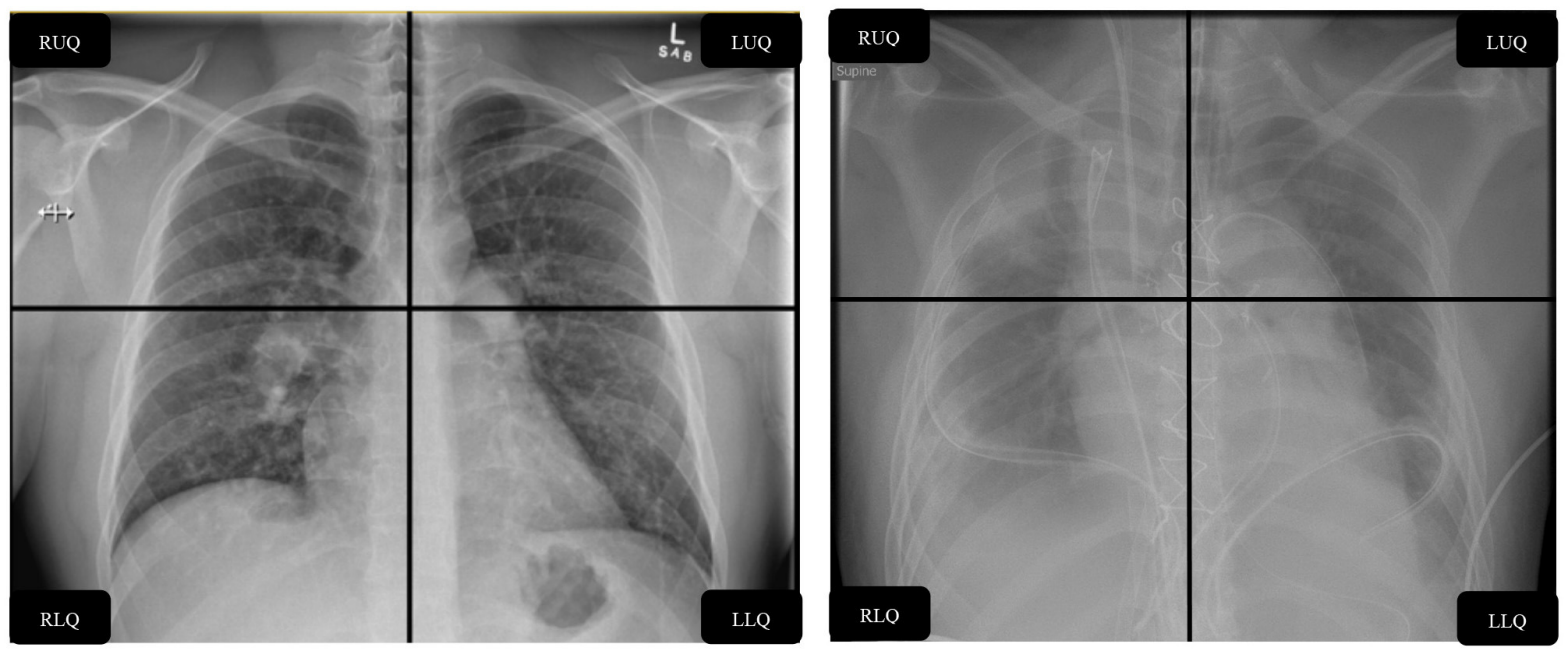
Example calculation of RALE score. RALE, radiographic assessment of lung edema; RUQ, right upper quadrant; RLQ, right lower quadrant; LUQ, left upper quadrant; LLQ, left lower quadrant; CXR, chest X-ray; ECMO, extracorporeal membrane oxygenation.
